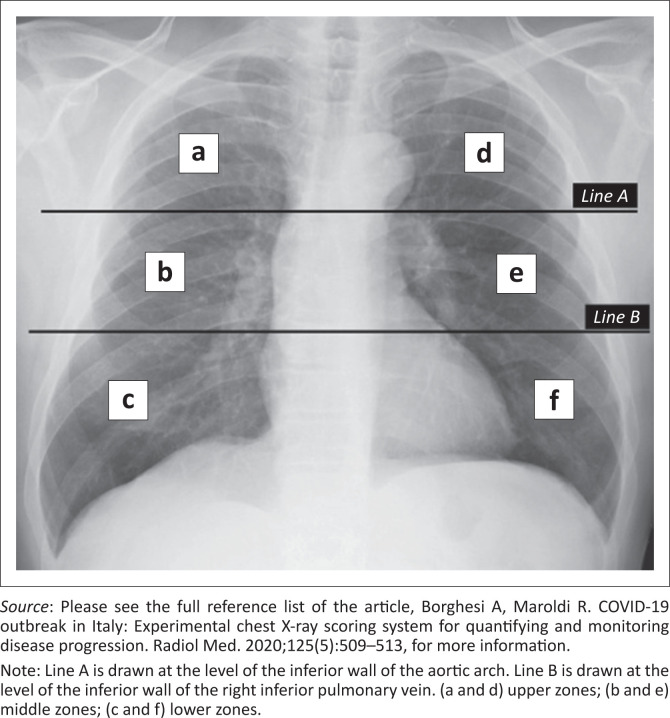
Division of lungs into six zones on frontal chest radiograph.
